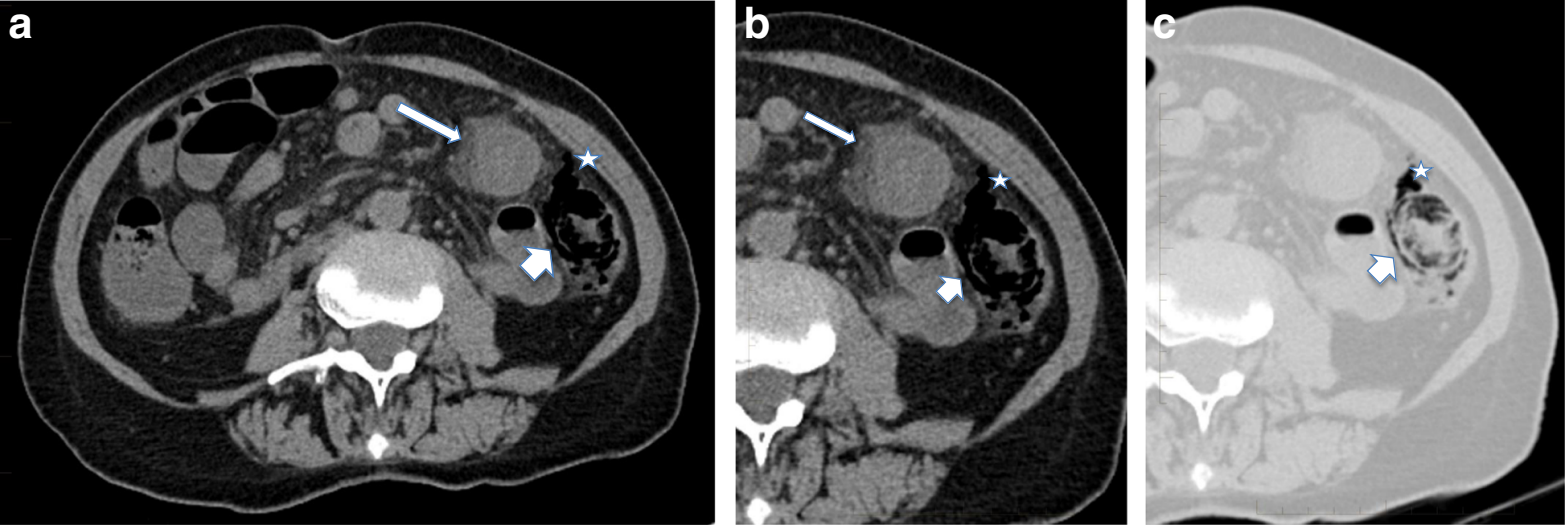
Radiologic images: a Axial view of unenhanced abdominal CT showing thickened bowel wall with fat stranding (*thin arrow*) involving the colonic splenic flexure. b and c Zoomed axial view of the left abdomen in soft tissue (b) and pulmonary (c) windows showing pneumatosis (*thick arrow*) and free air in the surrounding mesocolon (*star*)
